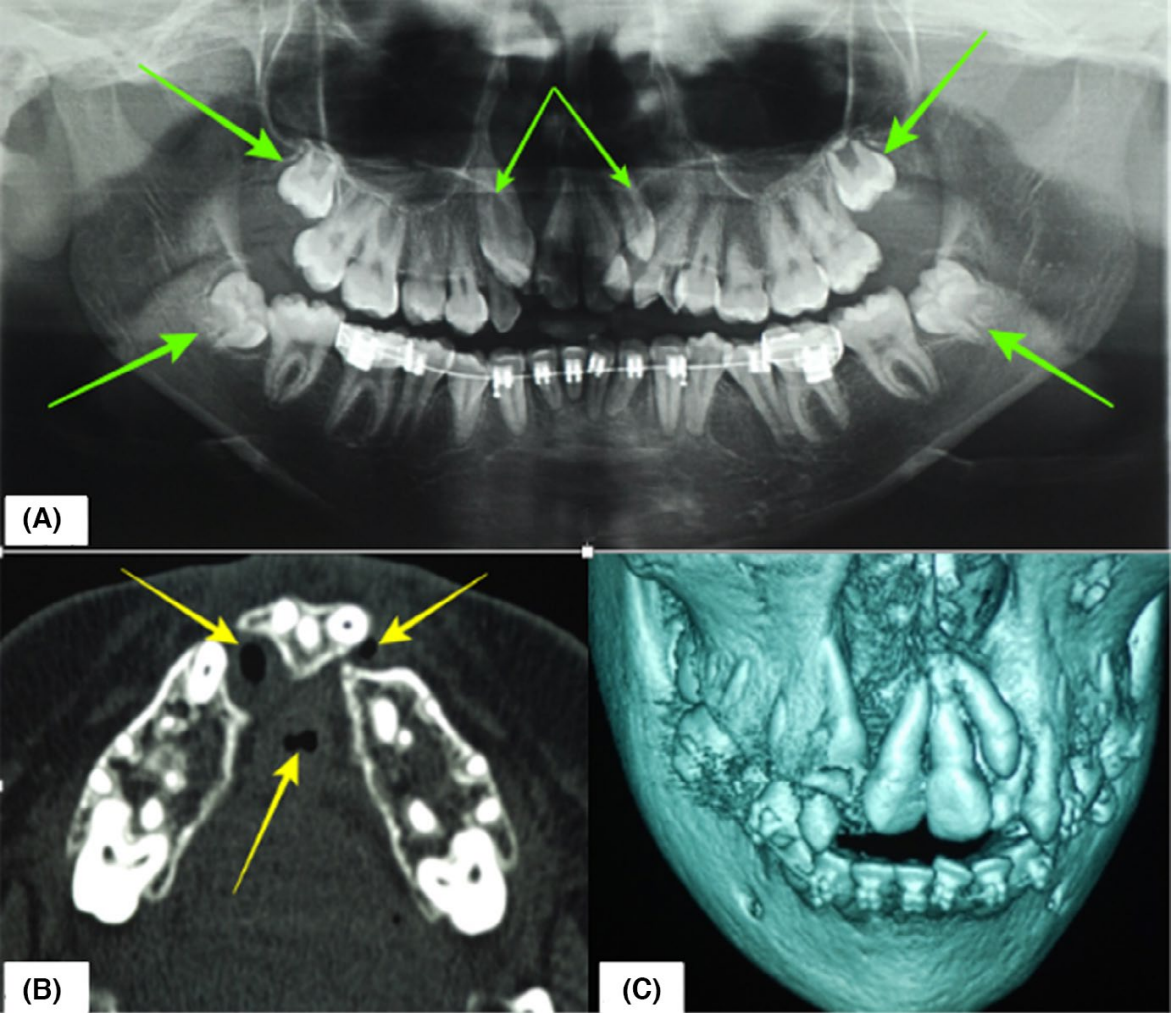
(A) Panoramic radiograph at the beginning of treatment showing anterior maxillary crowding with impacted teeth (green arrows). (B) Axial section CT scan revealing a discontinuity of the maxillary alveolus accompanied by fistulae (yellow arrows). (C) A 3D reconstruction showing the floating anterior maxillary segment
